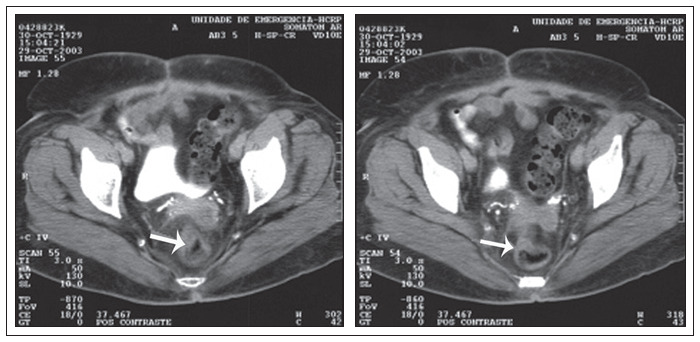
Computed tomography of the abdomen and pelvis of a 74-years-old woman, showing parietal thickening in the rectosigmoid transition.
